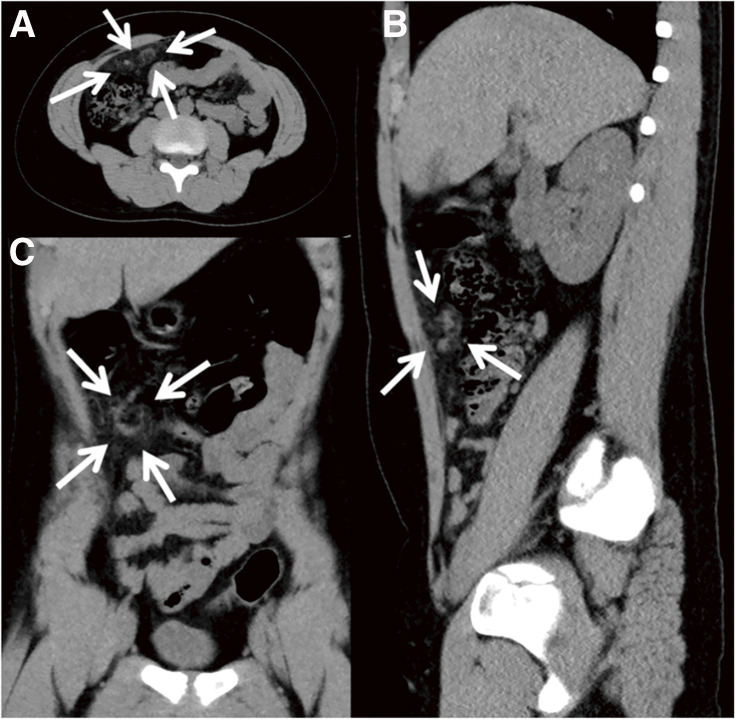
CT results of Case 2. (A) Transverse plane. (B) Sagittal plane. (C) Coronal plane. Whirl sign of omental torsion and increased fat concentration on CT (white arrows). CT, computed tomography
